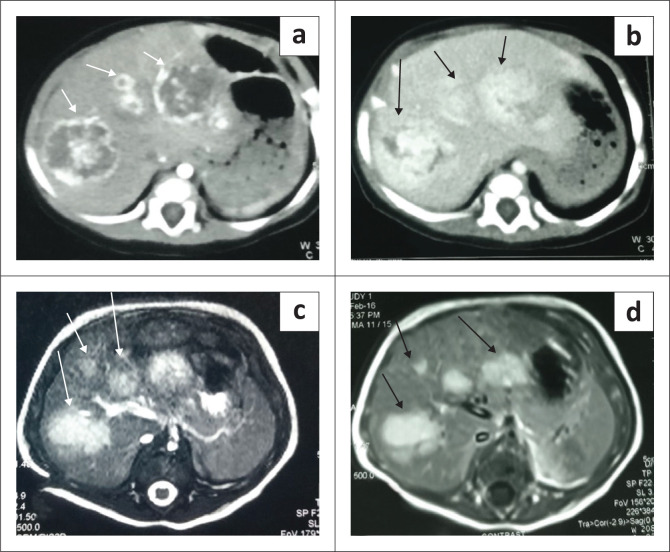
A 2-month-old female with multifocal infantile haemangioma. Multiphase CT (a and b) and MRI (c and d) through the liver show multiple focal lesions in both the lobes of the liver with peripheral nodular enhancement on the arterial phase (arrows in a) and progressive centripetal enhancement with near-complete filling on the delayed phase (arrow in b). Axial T2-weighted (T2W) image shows multiple hyperintense lesions in both the lobes of liver (arrows in c) and delayed phase post-contrast axial T1 MRI of the same patient shows near-complete filling similar to the CT scan (arrows in d).
